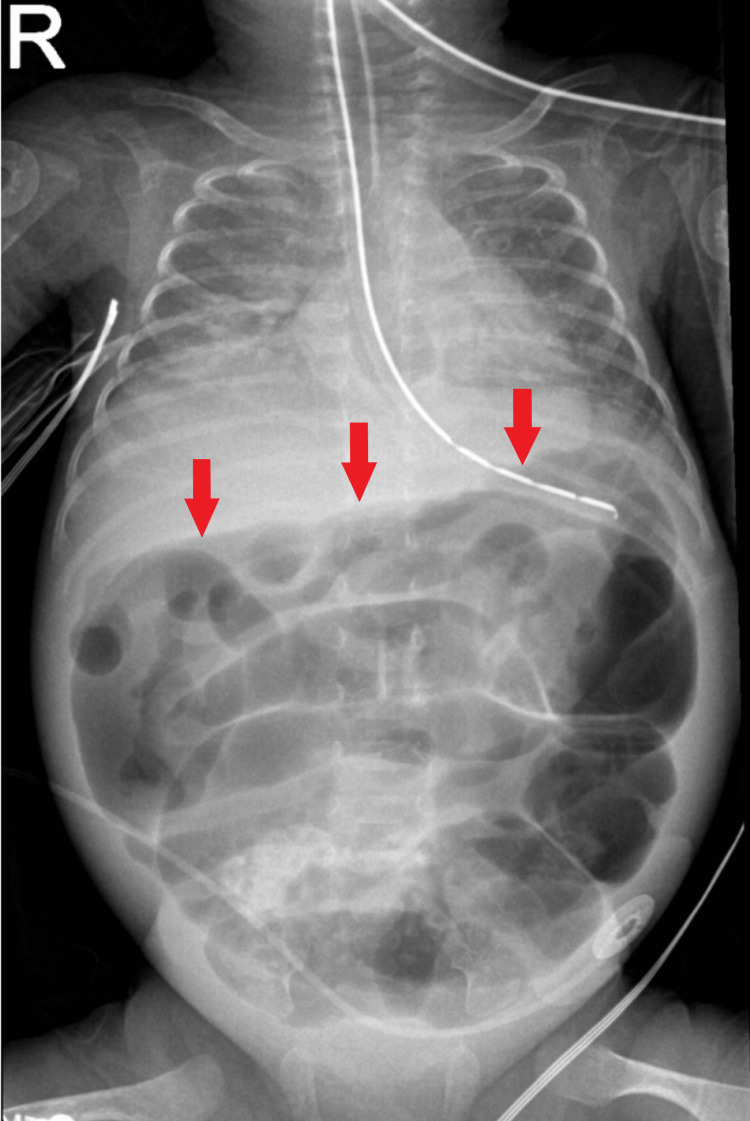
Antero-posterior infant X-ray demonstrating gaseous distention of bowel loops without pneumatosis or portal vein gas on day 47.
